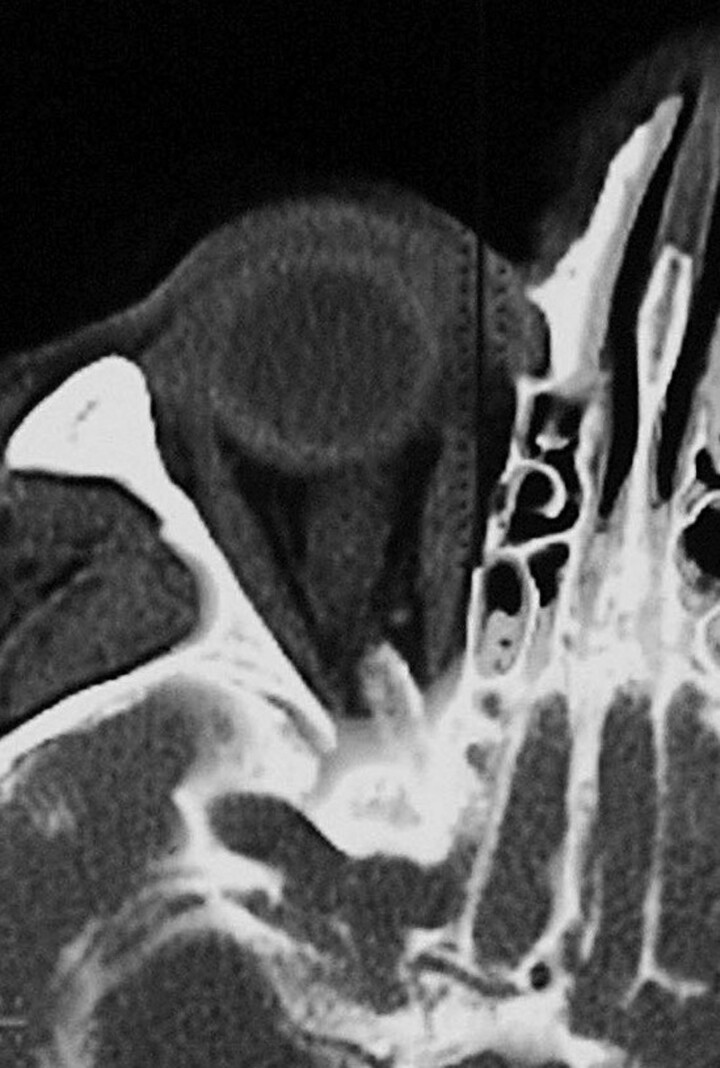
Computer tomography assisted cisternography. CT-assisted cisternography shows no flow of contrast medium into the intraorbital ON SAS in one of the included patients with idiopathic IIH and papilloedema.
